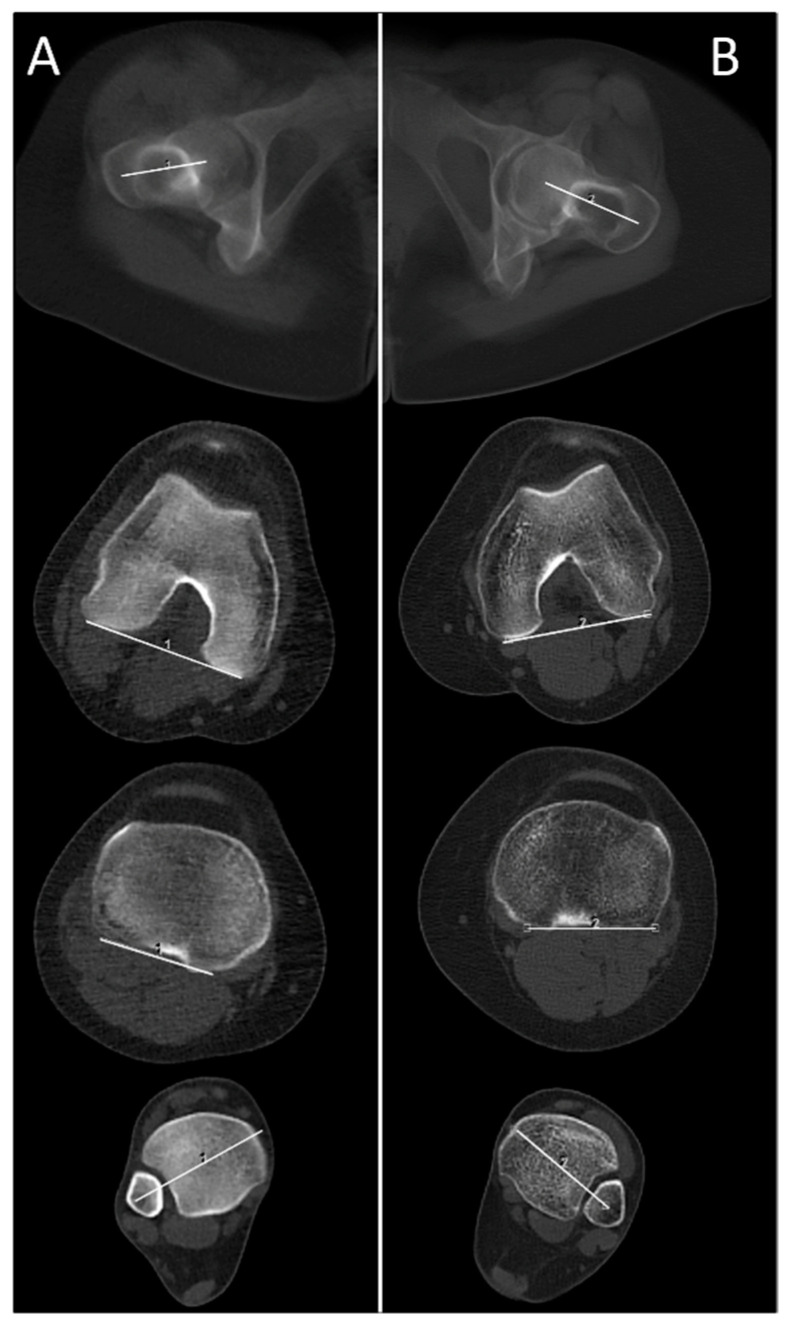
Comparison of a patient (A); female, 17 years, BMI = 25.2 kg/m^2^, no metal implants, total dose equivalent = 0.19 mSv examined with the newly introduced ultra-low dose (ULD) study protocol and a patient (B); female, 37 years, BMI 23.8 kg/m^2^, no metal implants, total dose equivalent = 4.09 mSv examined with the previous standard high-quality (HQ) study protocol for CT-torsion measurement (BMI = body mass index).
